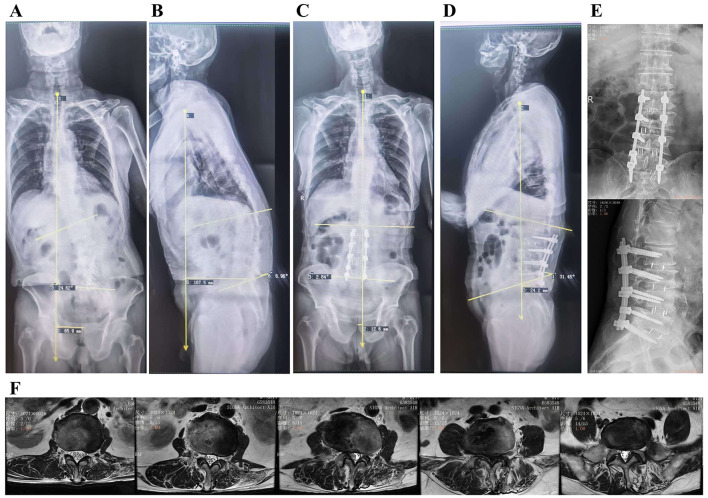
Pre-operative and post-operative radiographic and MRI findings. (A) Standing full-length anteroposterior radiograph of the spine demonstrating left lumbar scoliosis with a Cobb angle of 24.62° and coronal imbalance with 65.9 mm rightward deviation. (B) Standing full-length lateral radiograph of the spine showing loss of lumbar lordosis with kyphotic deformity, Cobb angle of 6.96°, and sagittal imbalance with 167.5 mm anterior translation. (C) Standing full-length anteroposterior radiograph showing excellent correction of scoliosis with well-positioned instrumentation, Cobb angle improved to 2.84°, and coronal balance restored with 12.6 mm rightward deviation. (D) Standing full-length lateral radiograph demonstrating restoration of sagittal alignment with Cobb angle of 31.45° and improved sagittal balance with 24.2 mm anterior translation. (E) Anteroposterior and lateral lumbar radiographs at 24-month follow-up showing well-positioned interbody cages and instrumentation with no screw loosening, and evidence of callus formation in the grafted regions indicating solid fusion. (F) Axial T2-weighted MRI of the lumbar spine demonstrating fatty infiltration of the left multifidus muscle with Goutallier grades ranging from 1 to 4 across the L1–2 through L5-S1 levels; erector spinae muscle showing Goutallier grades ranging from 2 to 4 across the L1–2 through L5-S1 levels.
